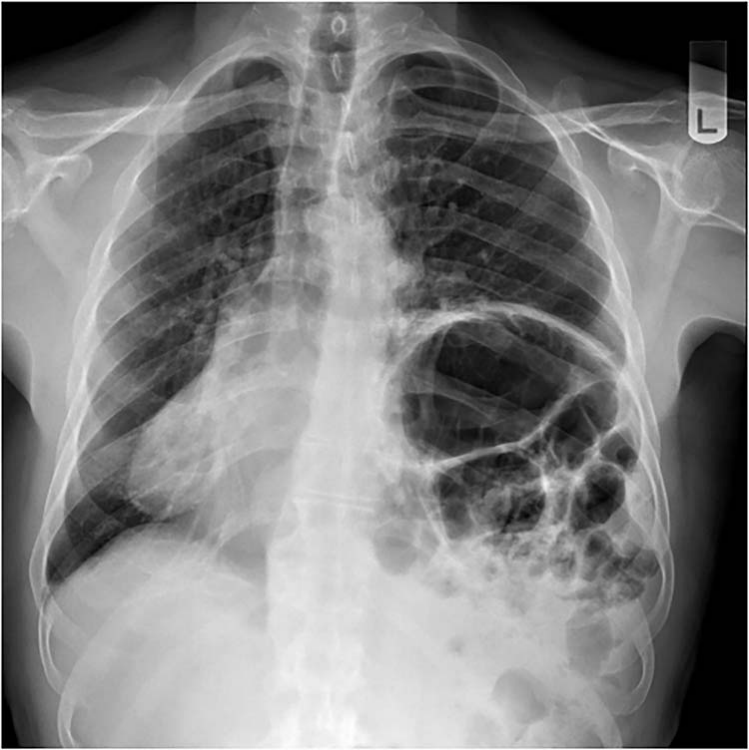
Chest X-ray performed preoperatively.
